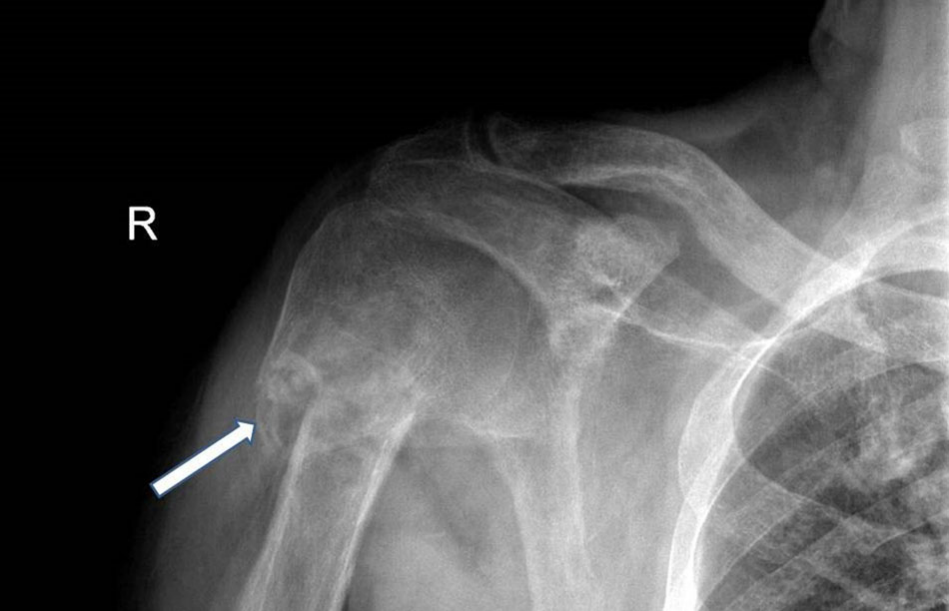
Case 3: x-ray of the pathological fracture, within extensive ostelytic lesion of right proximal humerus (arrow)
